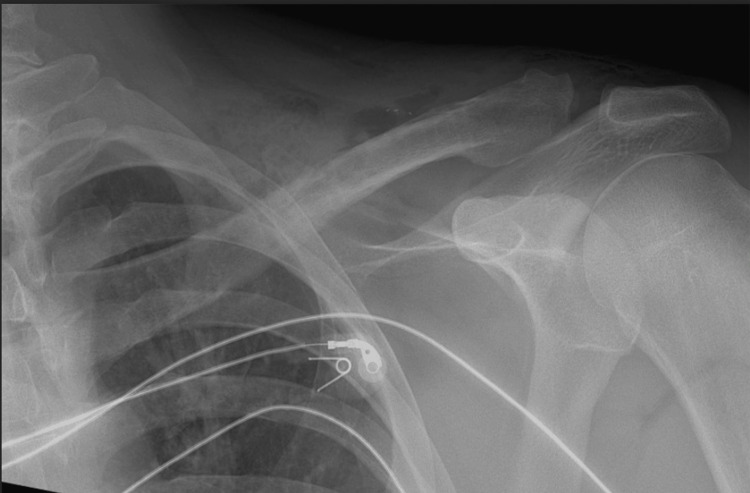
Post-implant removal radiograph showing no retained hardware.
